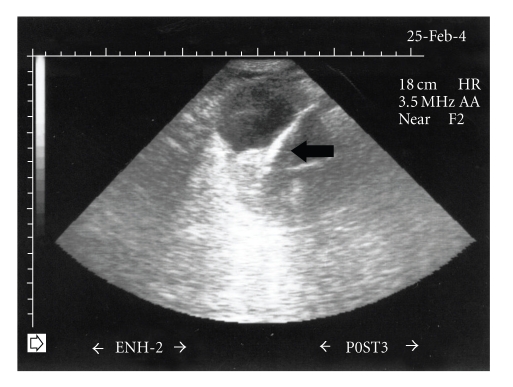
Ultrasonography shows a straight echogenic foreign body (arrow).
